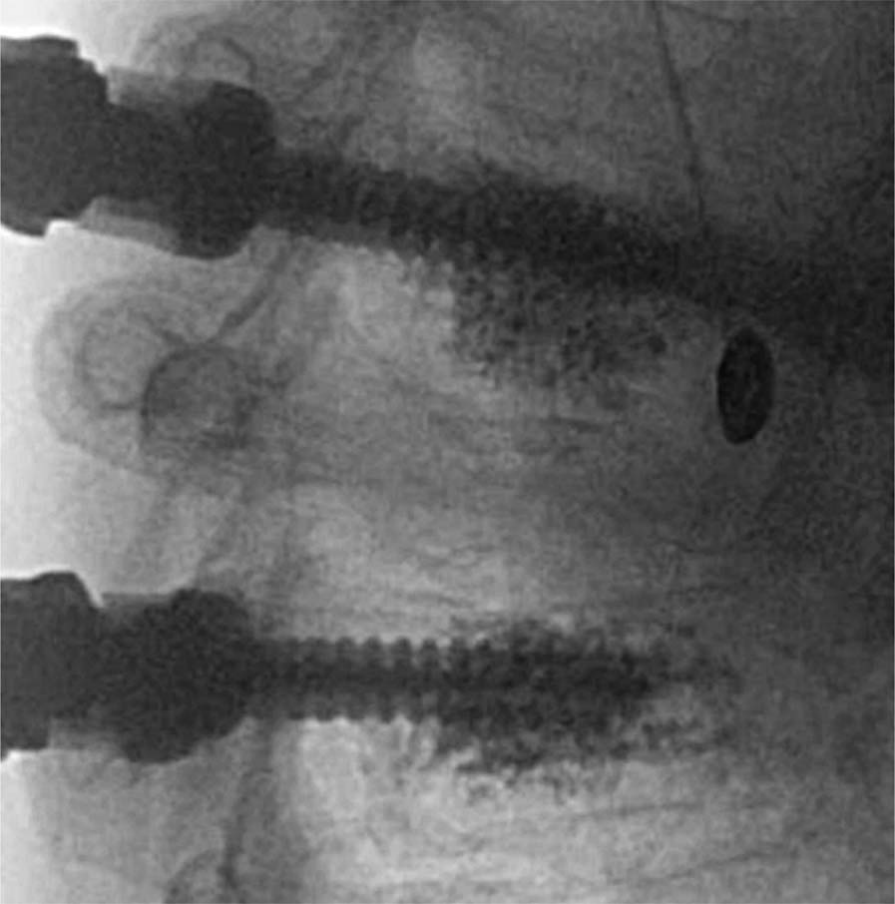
Lateral x-ray augmentation of a fenestrated screw, note the wider distribution of the polymethylmethacrylate around the vertebral body.
